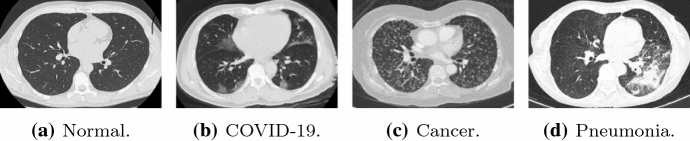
Samples of four lung CT scans drawn from the used training and test data sets
